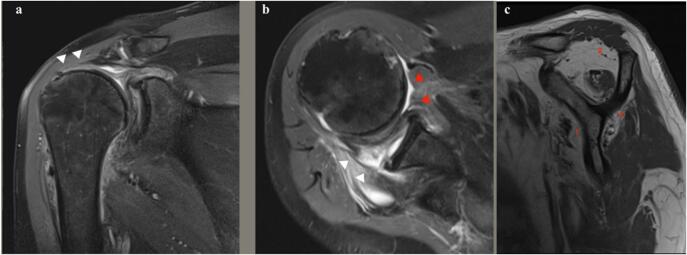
Magnetic resonance imaging of the right shoulder; (a) T2 Coronal view: Tear of the supraspinatus muscle is observed (white arrow) with retraction of the tendon to the level of the glenoid. (b) T2 Axial view: Tear of both the subscapularis tendon (red arrow) and infraspinatus tendon (white arrow) can be observed, with significant retraction of the tendon almost to the level of the glenoid. Anterior subluxation of the shoulder can be observed. (c) T1 Sagital view, significant fatty infiltration of the subscapularis (1), Supraspinatus (2) and Infraspinatus (3) muscles can be observed. (For interpretation of the references to colour in this figure legend, the reader is referred to the web version of this article.)
